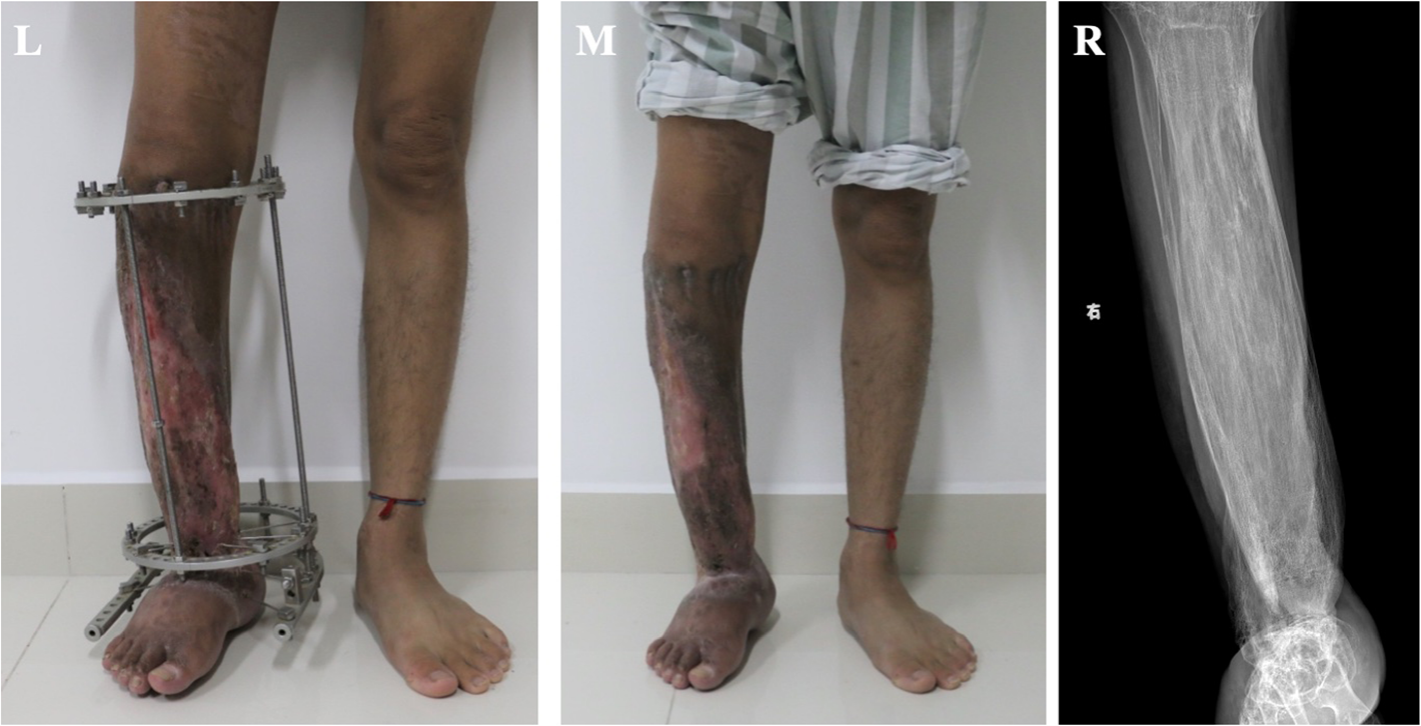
L, photo of bilateral lower legs at completion of distraction. M, photo of bilateral lower legs after removal of apparatus. R, X-ray of newly formed right tibia after removal of apparatus
